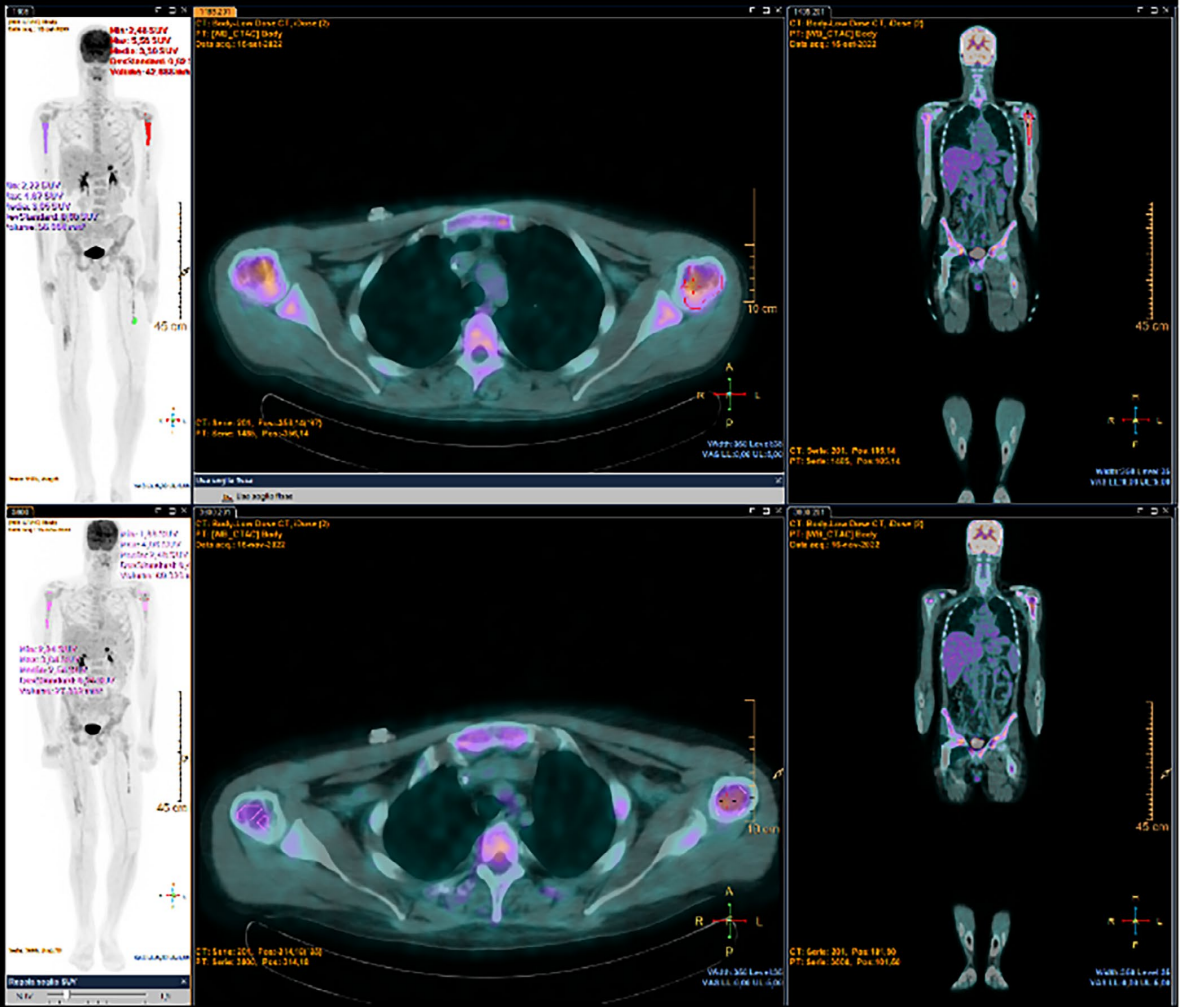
PET‐CT performed before (a) and after (b) three cycles of HD‐ifosfamide, showing partial metabolic response to treatment on bone marrow infiltration.
